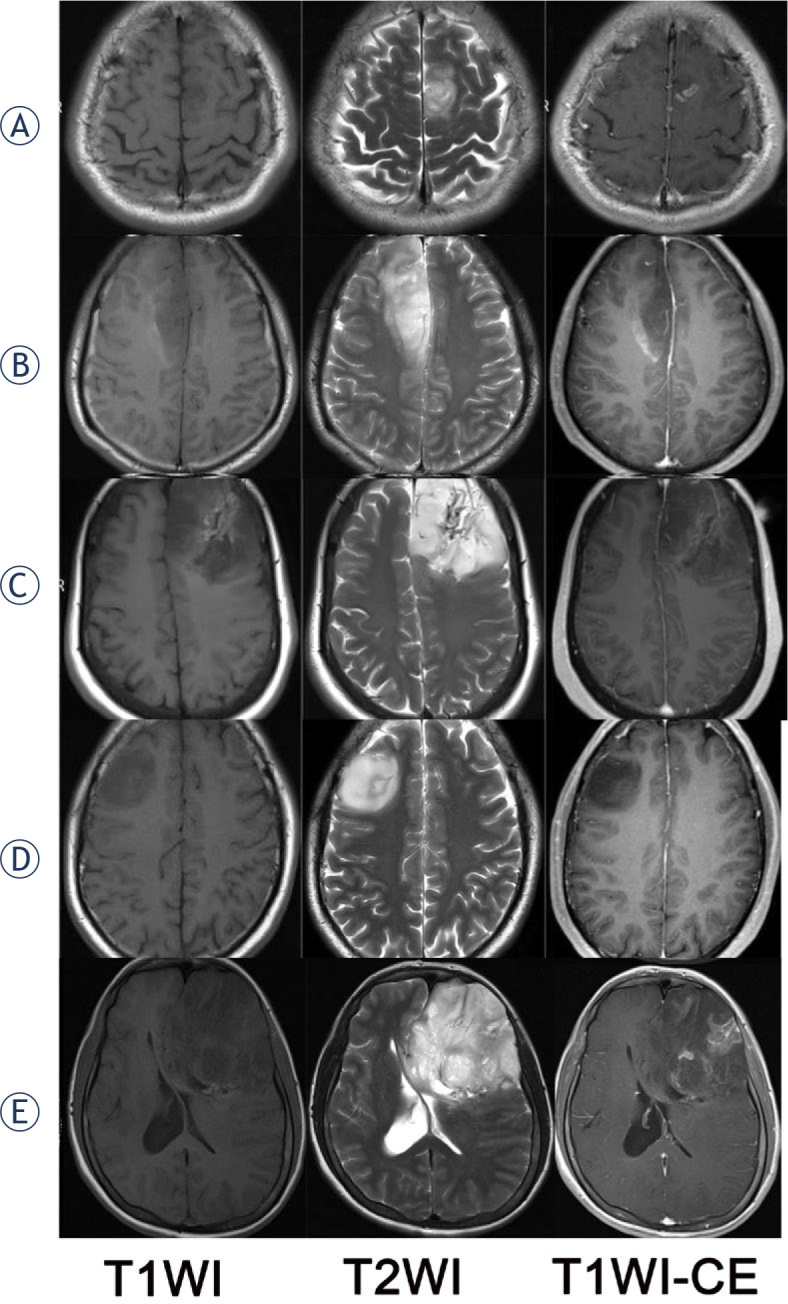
Some representative MRI images without imaging necrosis which was exactly confused in diagnosing imaging necrosis. Shown from left to right by the order are T1WI, T2WI, and T1WI-CE. (A) a 24-year-old man with an oligodendroglioma, IDH-mutant and 1p/19q-deleted, CNS WHO grade 2; (B) a 39-year-old man with an oligodendroglioma, IDH-mutant and 1p/19q-deleted, CNS WHO grade 2; (C) a 55-year-old woman with an oligodendroglioma, IDHmutant and 1p/19q-deleted, CNS WHO grade 2; (D) a 45-year-old man with an astrocytoma, CNS IDH-mutant, WHO grade 2; (E) a 36-year-old woman with an oligodendroglioma, IDH-mutant and 1p/19q-deleted, CNS WHO grade 2. In this case (E), it showed multiple long tubular and filiform enhancement and there were some tumor areas with remarked decrease of reinforcement. But these areas are hyperintense, not hypointense, on the T1-weighted image. Comparing with CT images (not provided), calcification on these areas were just observed. So, there was no imaging necrosis in these conditions.
